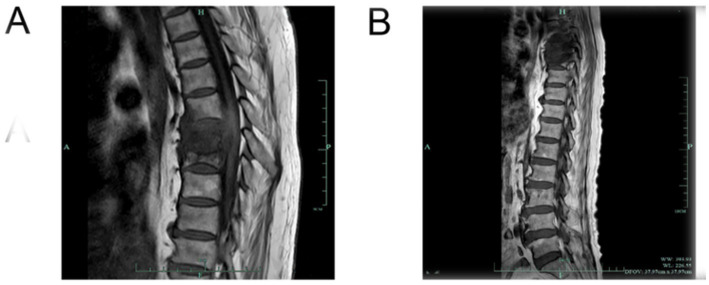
(A,B) Results of thoracic MRI before treatment.
